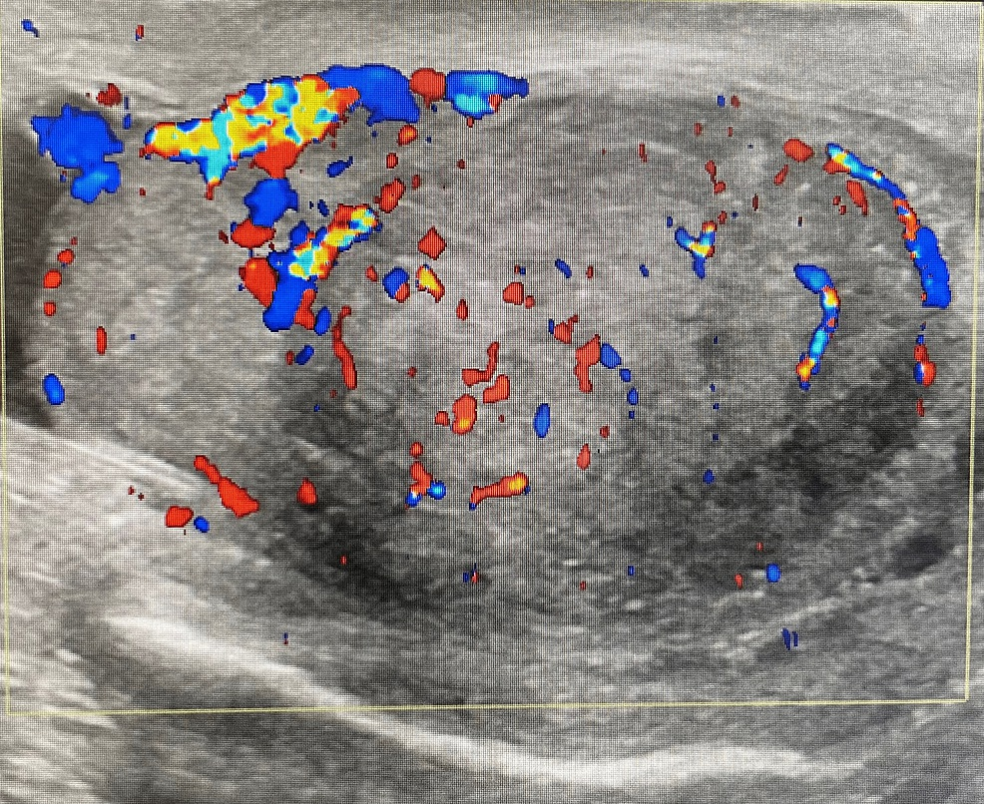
Scrotal ultrasound showing the right testis is enlarged, measuring 3.5 cm x 3.1 cm x 5 cm, demonstrating heterogeneous parenchymal echotexture occupied by a large vascular intratesticular heterogenous hypoechoic lesion measuring 3 cm x 3.6 cm x 3.4 cm. No calcification seen. The right epididymis appears to be swollen with normal vascularity.
